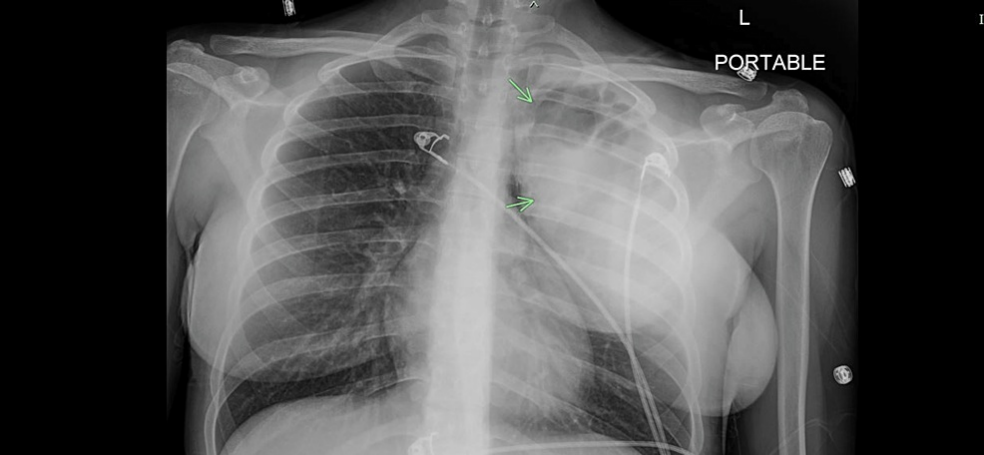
Chest X-ray on presentation showing the left-sided lung abscess
